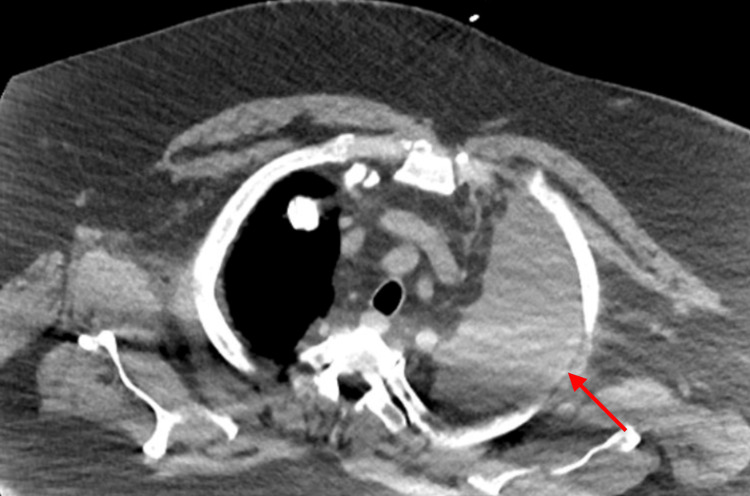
Axial chest CT at presentation demonstrating a large loculated left pleural fluid collection Axial computed tomography (CT) of the chest obtained at presentation demonstrates a large loculated left pleural collection (red arrow), consistent with empyema, with marked compressive atelectatic collapse of the adjacent left lung.
